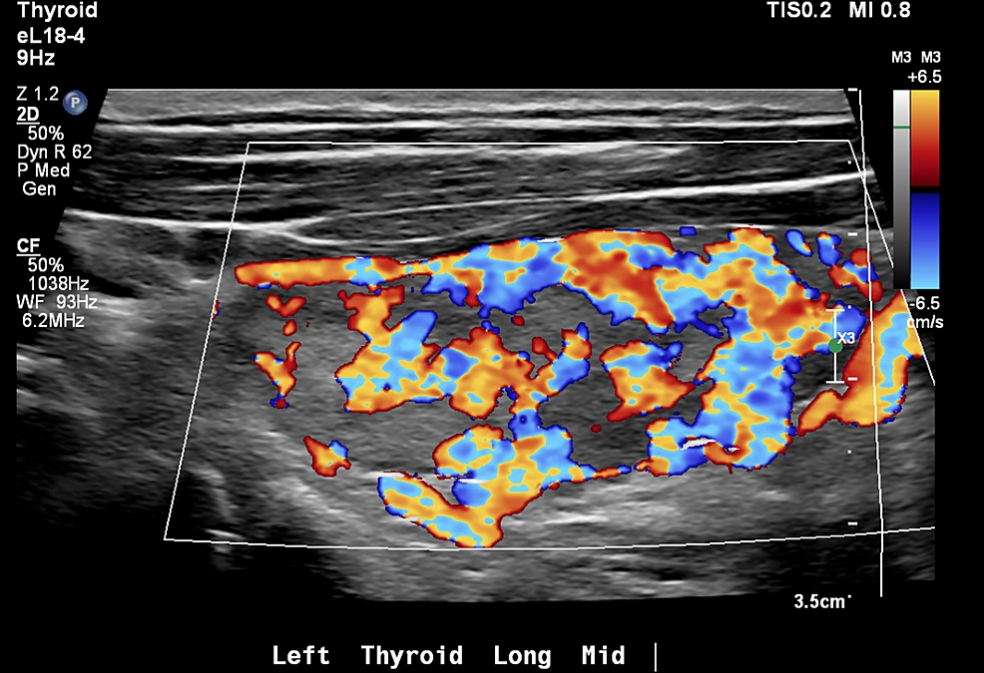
Increased flow of the left thyroid lobe on color doppler ultrasound
